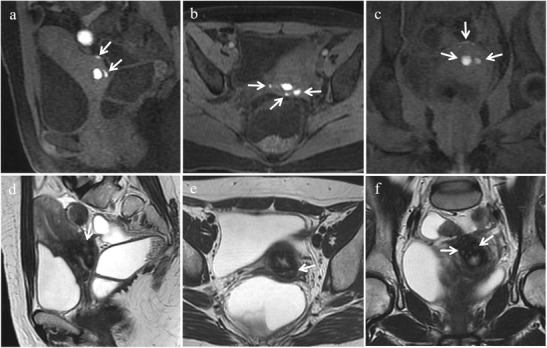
Endometriosis of the vagina and cervix in a 46-year-old woman. (a) Sagittal, (b) oblique axial, (c) oblique coronal fat-suppressed T1-weighted images, and (d) sagittal, (e) oblique axial and (f) oblique coronal T2-weighted images show small endometriotic implants involving the posterior vaginal fornix and the cervix, with multiloculated appearance (white arrows) characterized by cystic areas with hyperintense content, better depicted on fat-suppressed T1-weighted images due to subacute blood products
